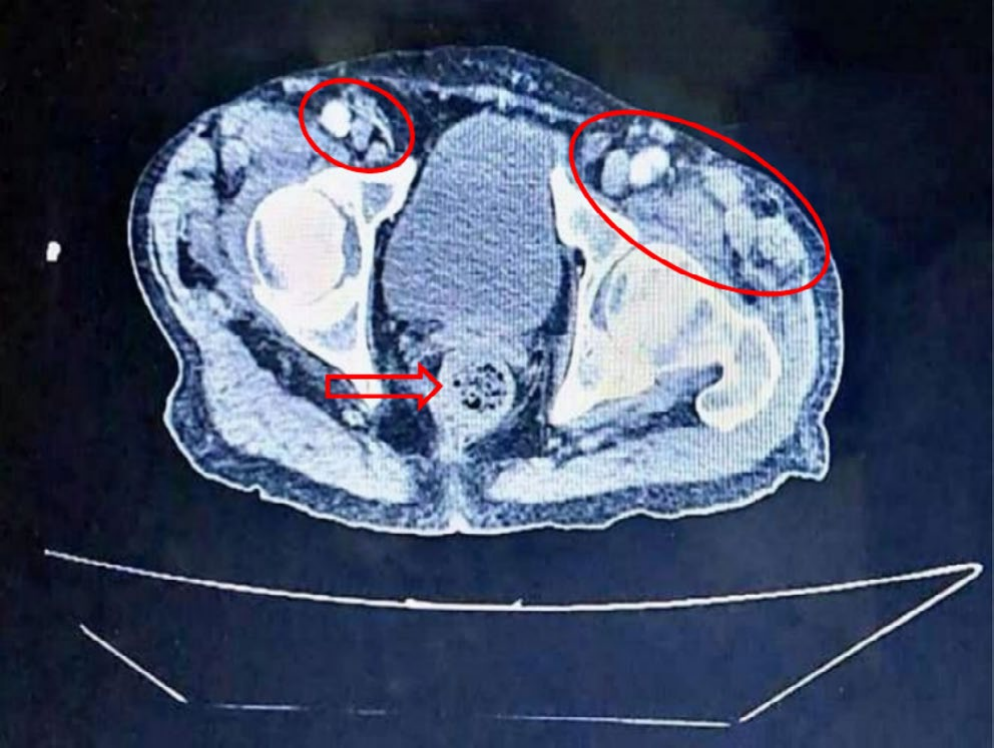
Pelvic CT scan Contrast‐enhanced pelvic CT scan of the pelvis demonstrating multiple enlarged pelvic lymph nodes (circle), the largest measuring 13 × 14 mm. Mild degenerative changes in the lumbar spine are also seen (arrows). Both findings are suggestive of reactive or metastatic involvement.
